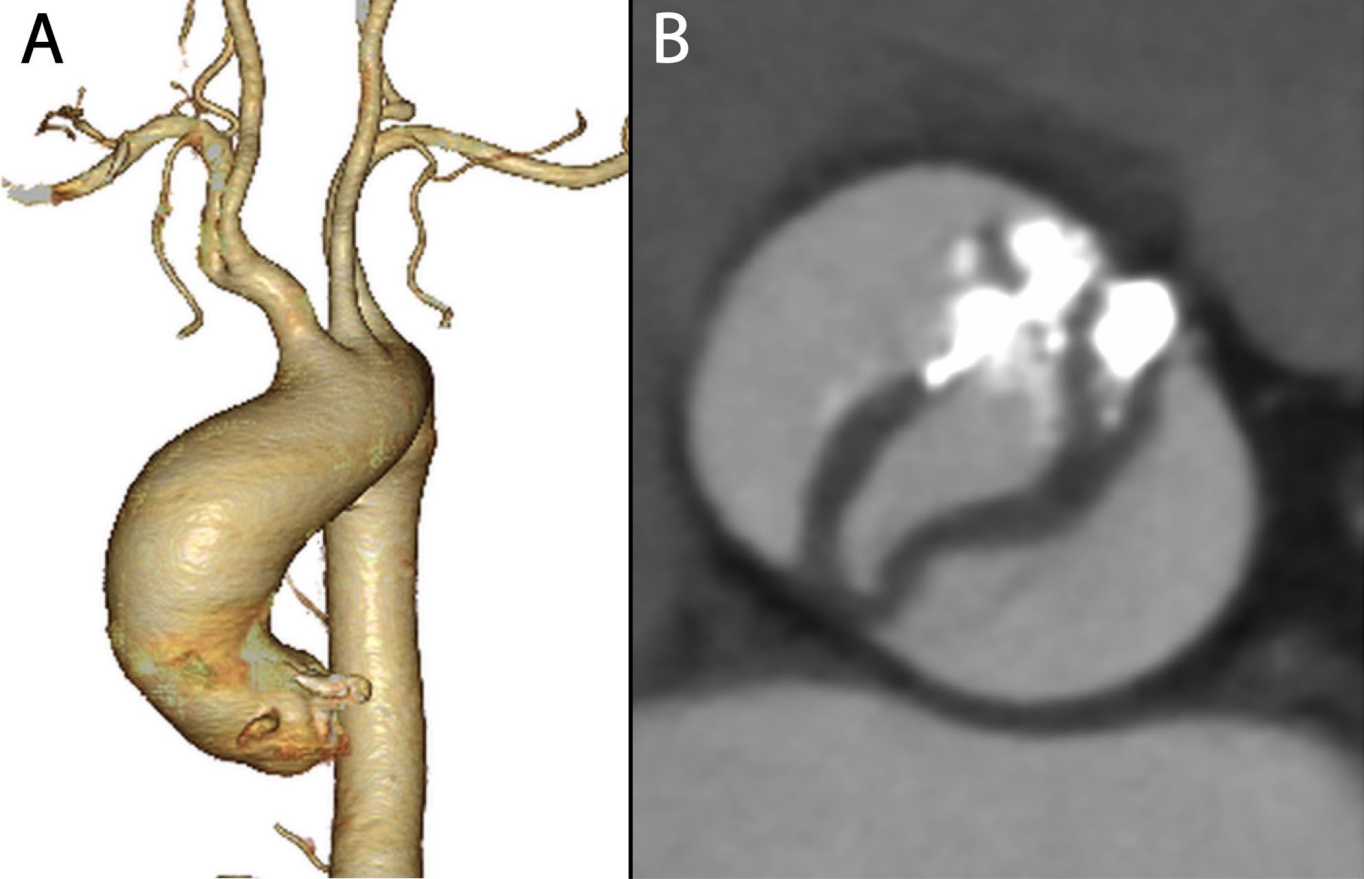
Computed tomography imaging before the operation. A Volume rendering image showing dilation of the ascending aorta. B Maximum intensity projection (MIP) image of the aortic valve demonstrating fusion of the non-coronary cusp and right coronary cusp, along with significant calcification at the commissure between the right coronary cusp and left coronary cusp
